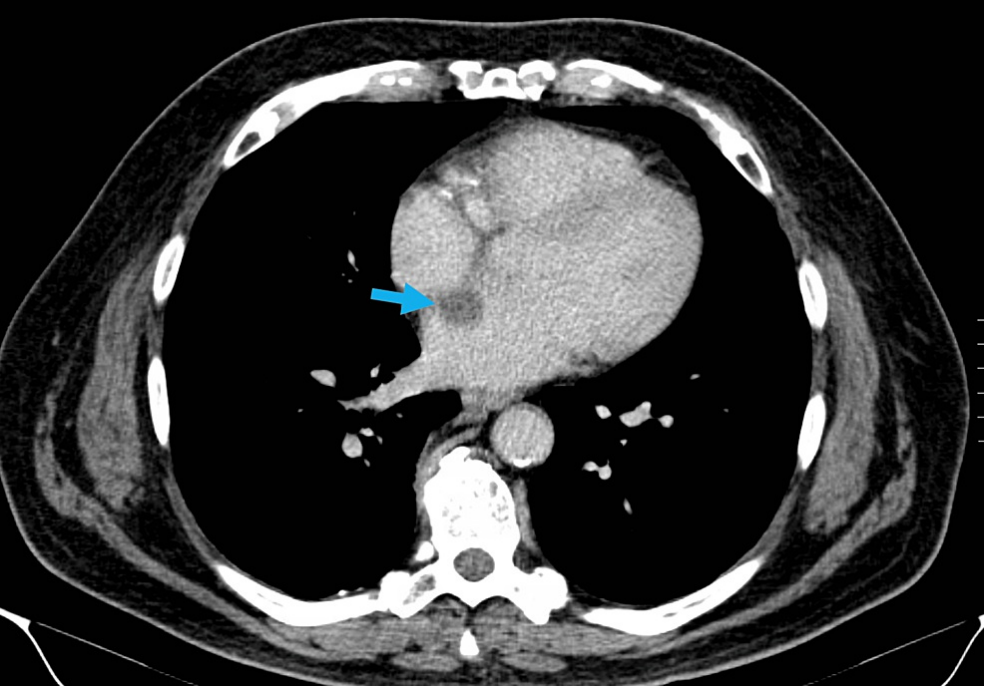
CT urogram showing an incidental partially visualized 2.1 cm round hypodensity extending into the left atrium from the interatrial septum (arrow). CT: computed tomography
